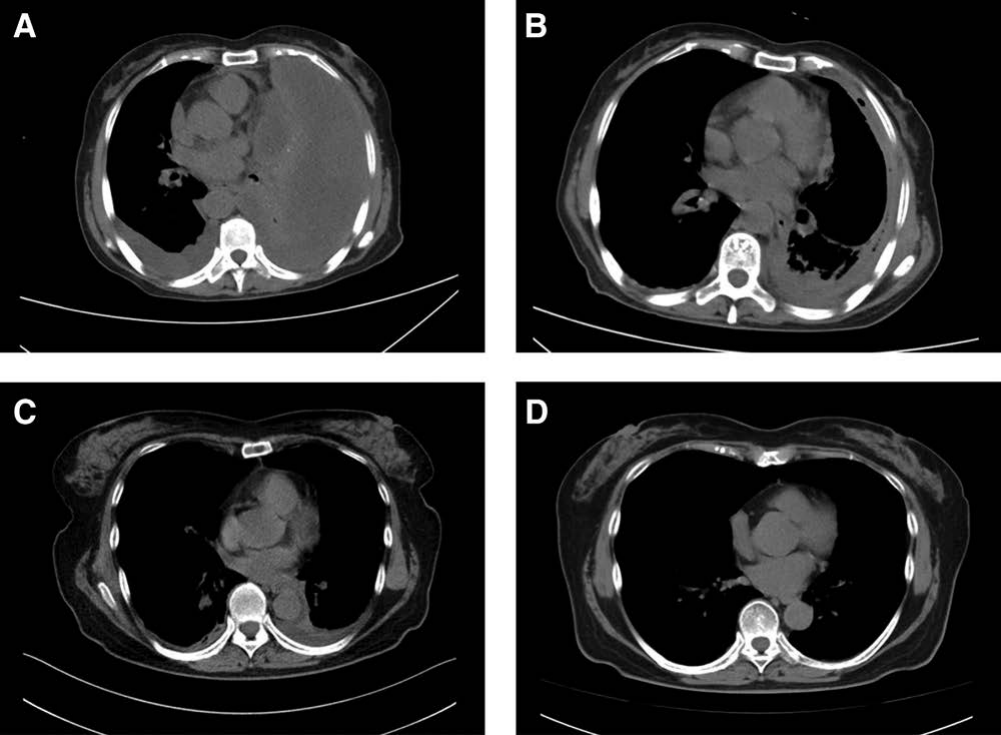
Chest CT findings in case No.1 and case No.2. Figure 1A shows the chest CT of case No.1 before treatment (January 10, 2023), and Figure 1B shows the chest CT after initiating antifungal treatment (January 26, 2023); Figure 1C shows the chest CT of case No.2 before treatment (December 17, 2022), and Figure 1D shows the chest CT after initiating antifungal treatment (January 26, 2023). CT = computed tomography.
